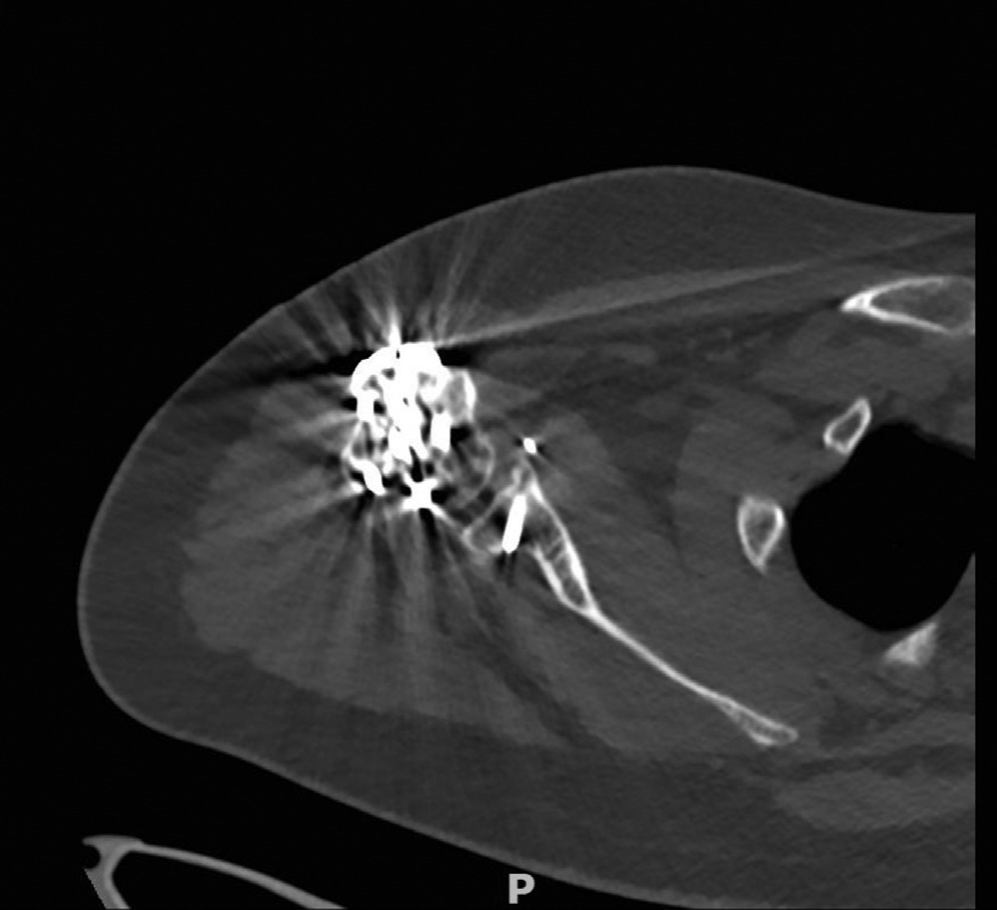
Another slide of the postoperative axial computed tomography scan after 8 months, showing complete union of the coracoid transfer and proximal humerus fracture.
